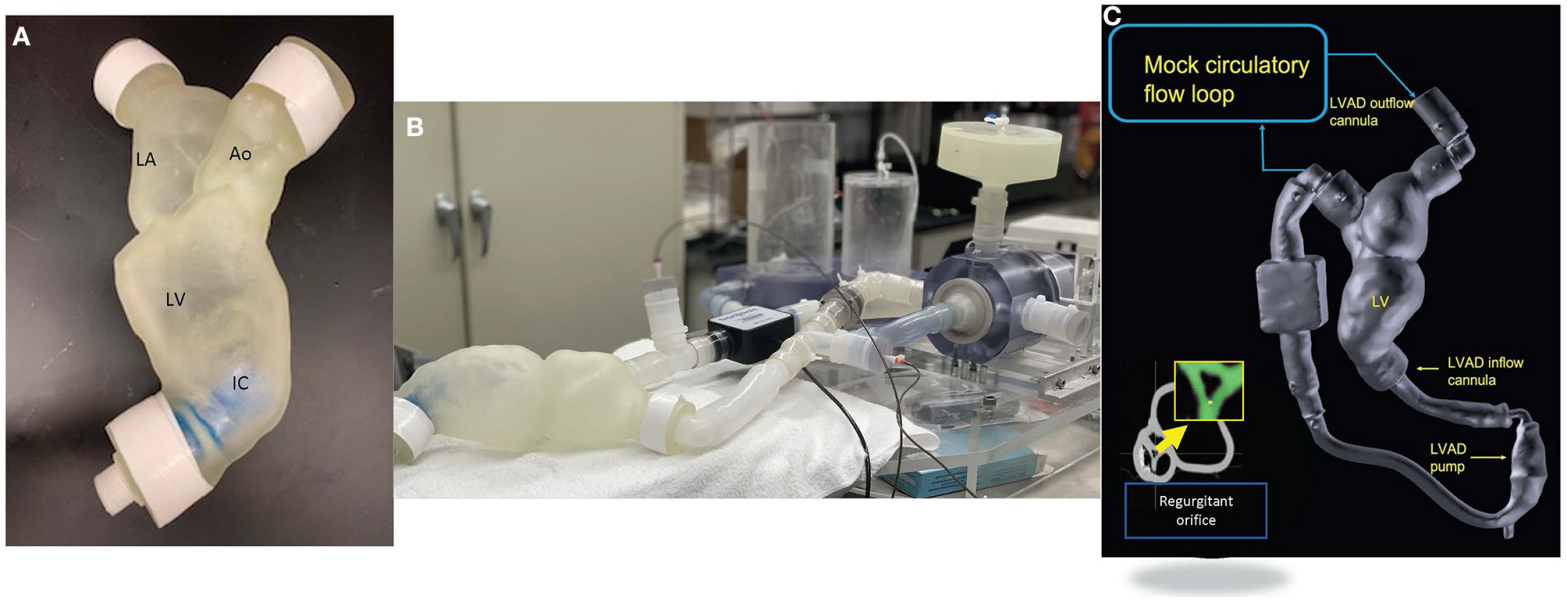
(A) Patient specific 3D printed model. (B) Mock circulatory flow loop setup. (C) Reconstruction from CT dataset of the patient specific model coupled with the Hearmate II LVAD and (inset) showing the regurgitate orifice. The yellow arrow indicates a zoom in on aortic regurgitation orifice.
